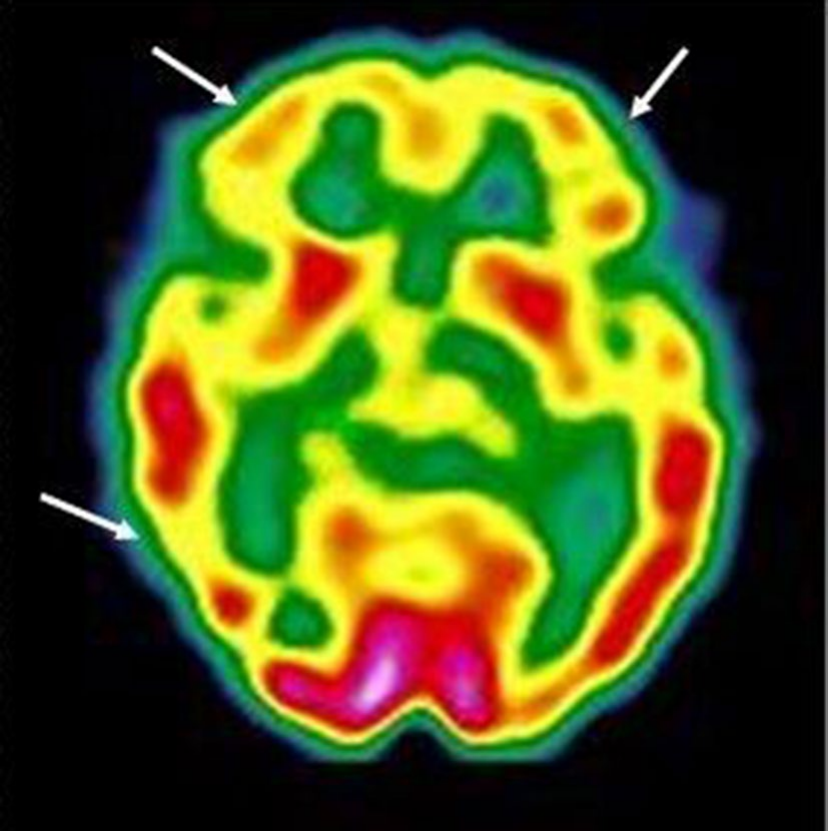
Case # 7. ^99m^Tc-ECD brain SPECT: decreased tracer uptake in both frontal lobes and in the right occipital region (*arrows*)
